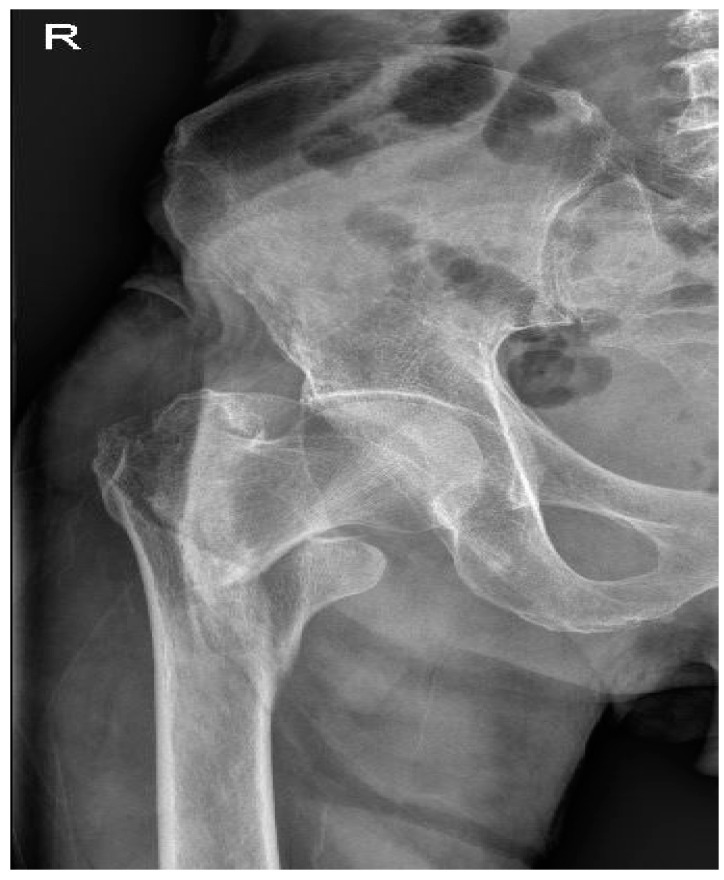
Fracture of the right hip femur intertrochanter (Pelvis AP (Anteroposterior) X-ray).
